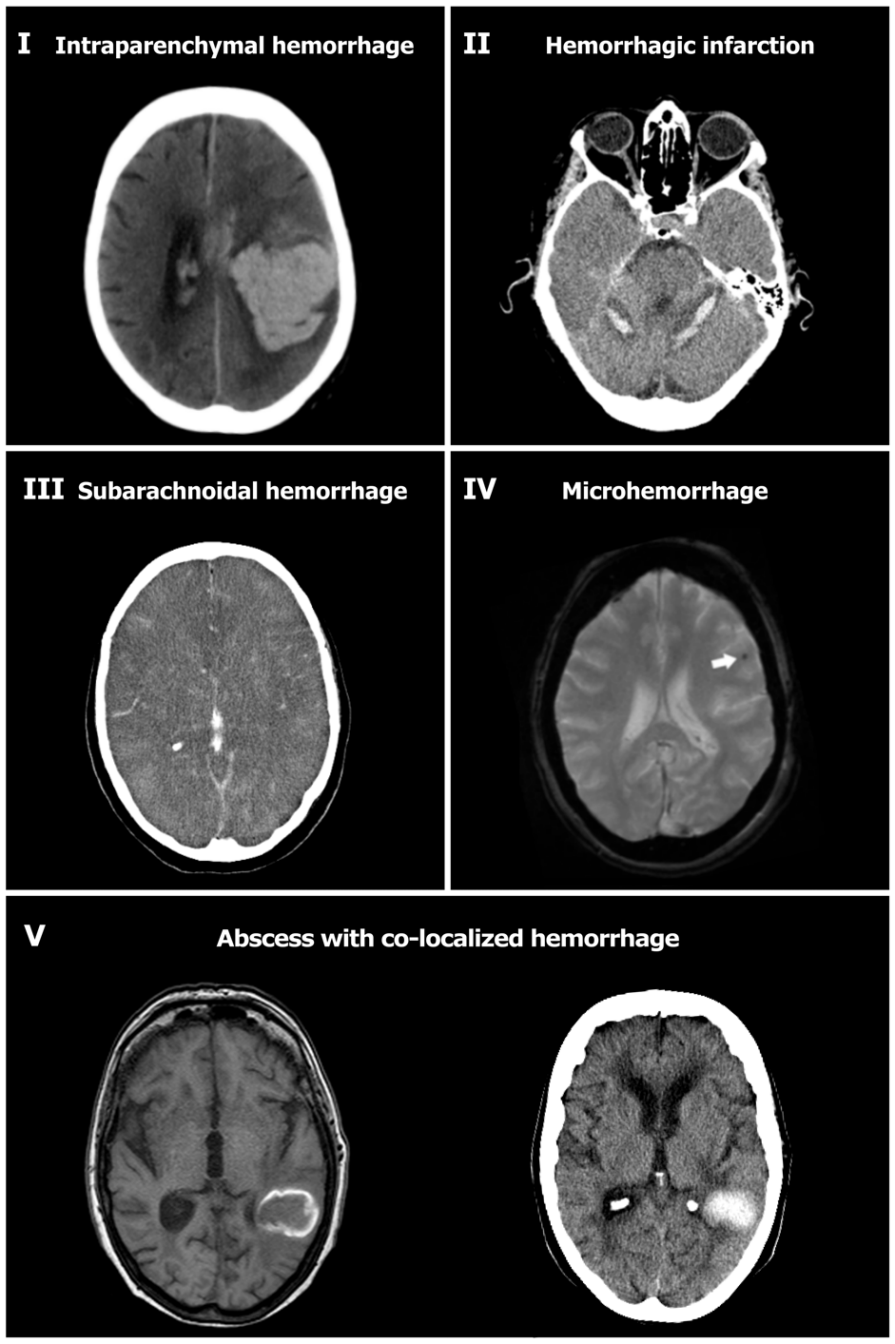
Types of intracranial hemorrhagic complications encountered in bacterial meningitis patients. Intraparenchymal hemorrhage in left parietal lobe (I); hemorrhagic infarction (II); subarachnoidal hemorrhage (III); micro-hemorrhages (IV, arrow depicts location of hemorrhage; MRI-gradient echo); Abscess formation and subsequent hemorrhagic transformation; panel V depicts MRI T-1 with gadolinium, and a CT-scan 5 days later).
